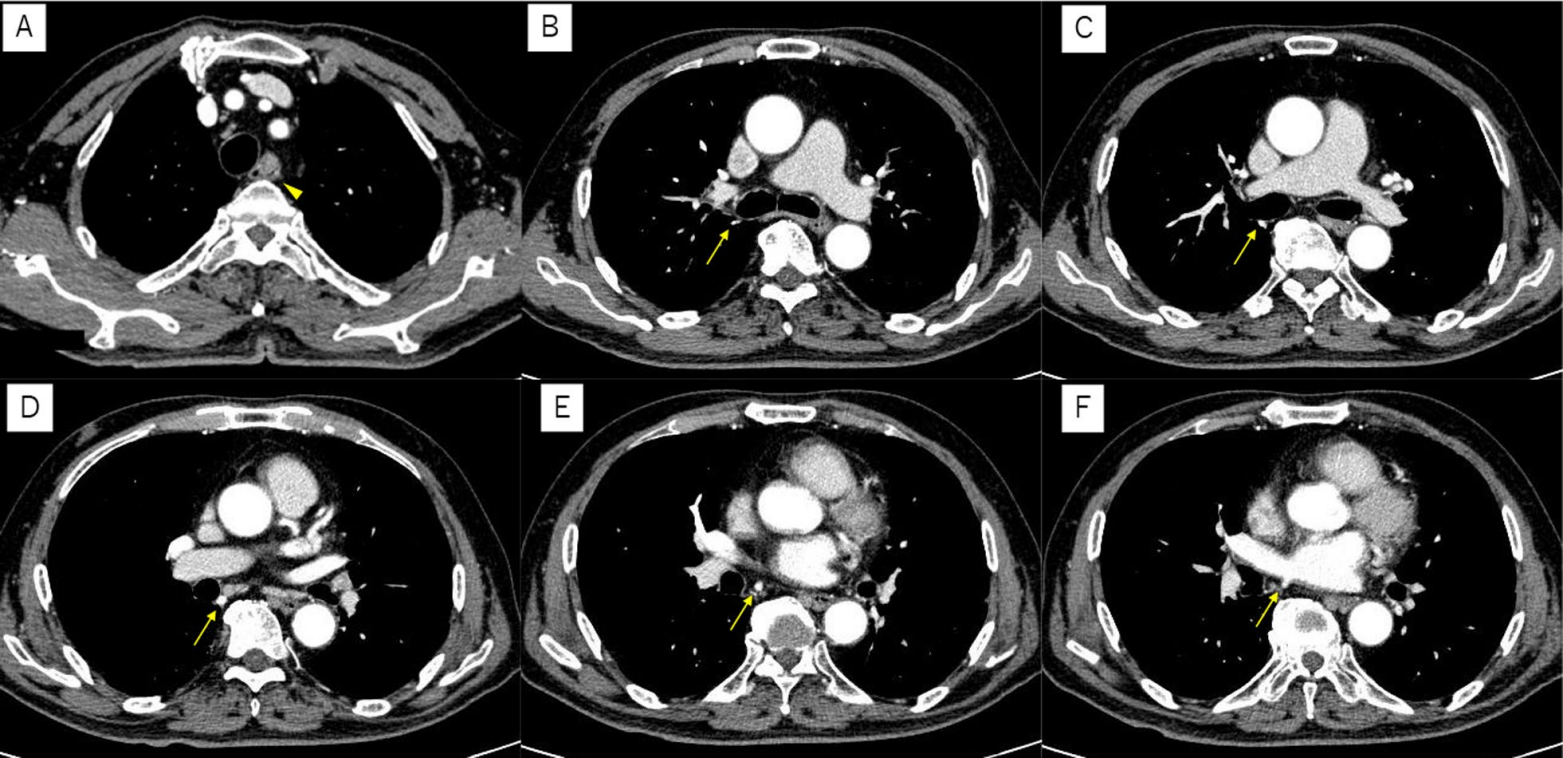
Contrast-enhanced CT findings. A Contrast-enhanced CT showed mural thickening of the anterior wall of the upper thoracic esophagus, with no evidence of multi-organ invasion or lymph node metastasis. B–F A break in the right pulmonary vein passing dorsal to the right main bronchus and flowing directly into the left atrium was observed (arrow in the figure)
